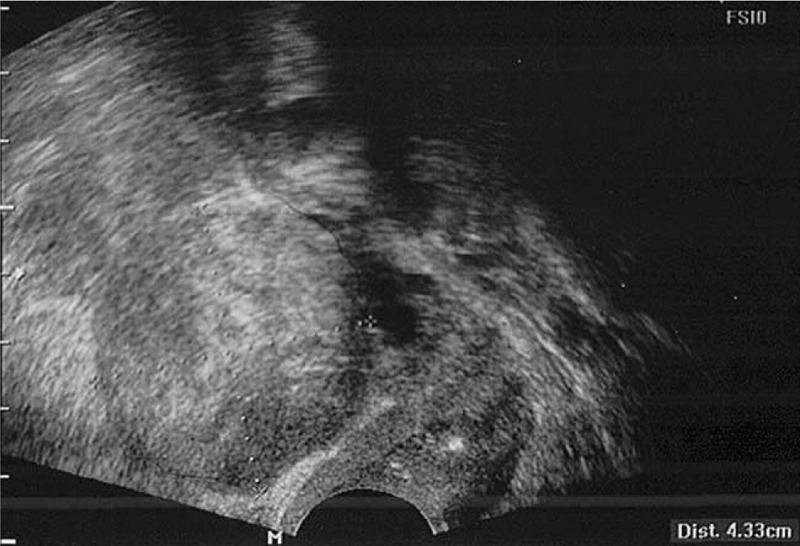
Transvaginal ultrasonography revealing a solid mass, measuring 4.3 cm × 4.3 cm, on the anterior wall of the uterus.
